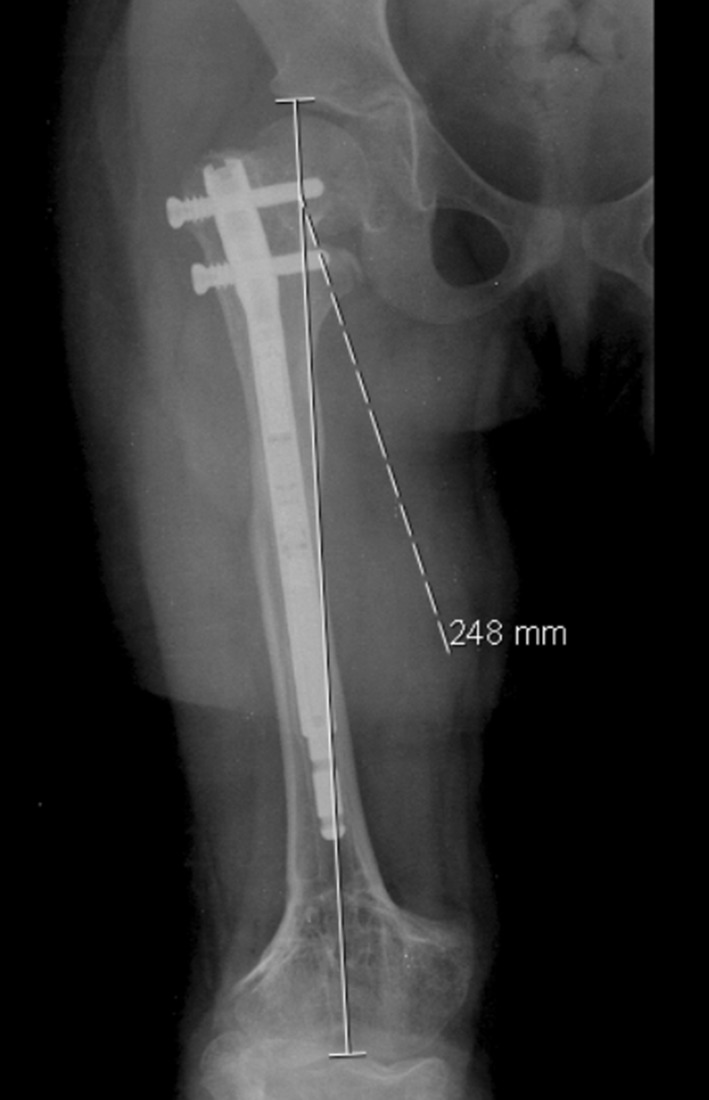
Postoperative X-ray after second surgery to remove distal locking screws
